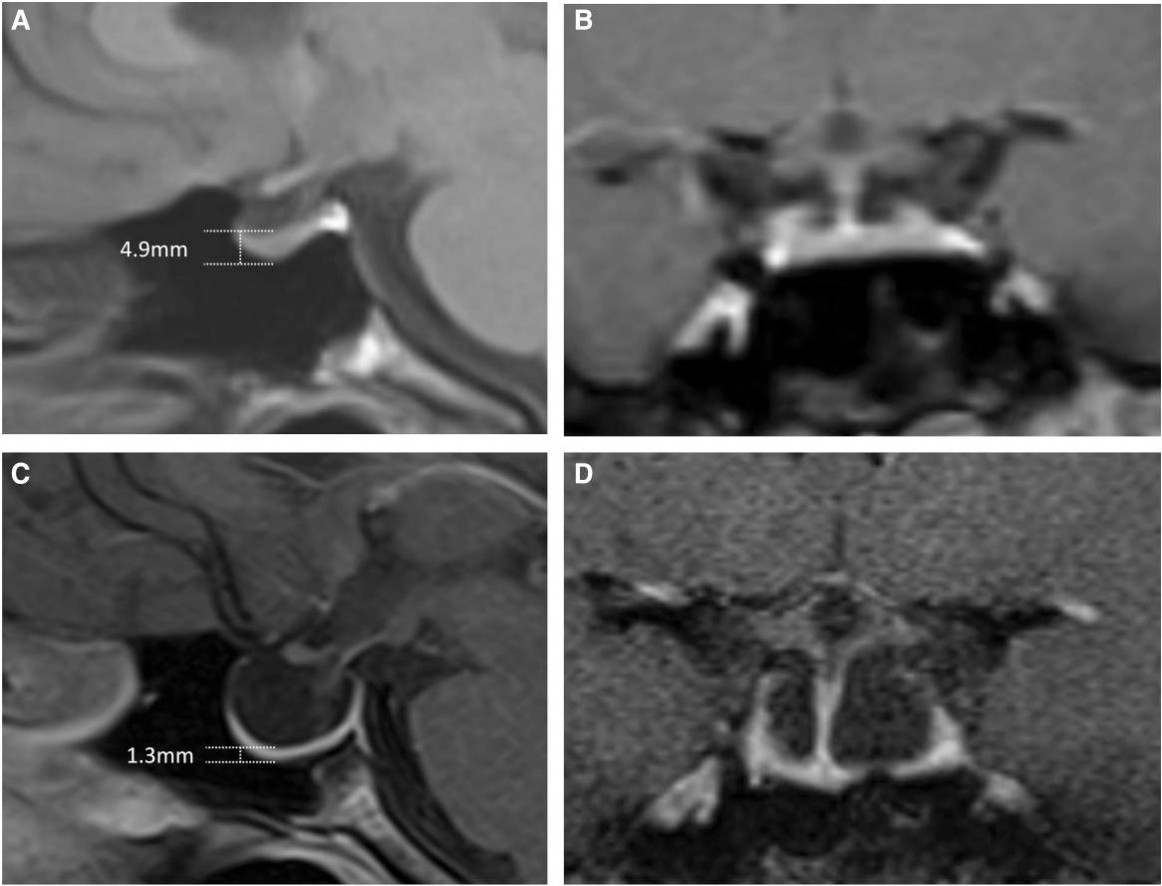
Sagittal and coronal views of pituitary gland magnetic resonance imaging at initial presentation (A-B) and after four years (C-D).
